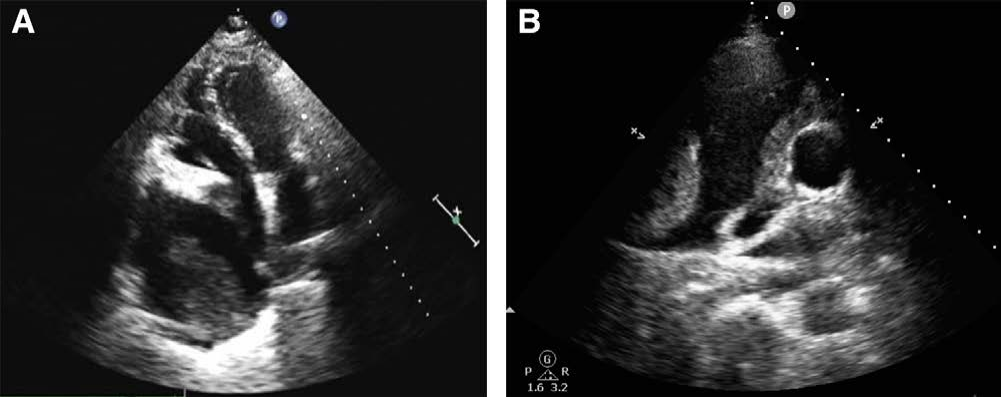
Echocardiogram. (A) Large amount of fluid accumulation in the right pericardium with solid echo mass inside, the size of which was about 54 * 71 mm, no obvious motion was observed. The right chamber was compressed and significantly deformed. (B) Irregular solid mass at the aortic root with sparse blood flow signals.
